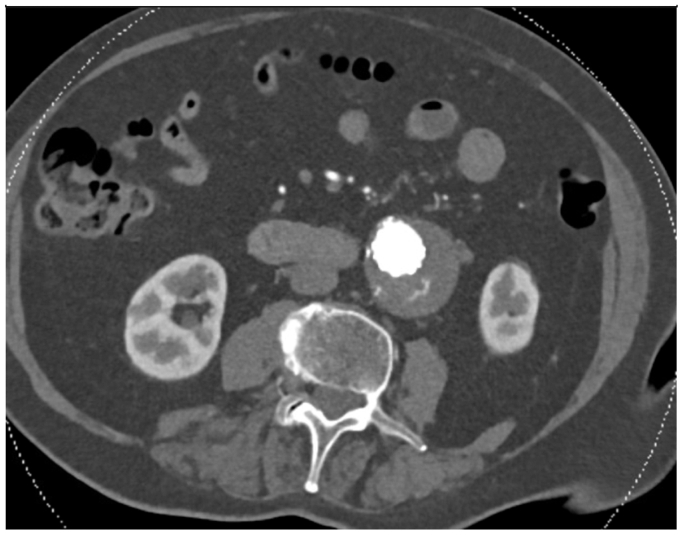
Computed tomography (CT) angiogram showing axial view of type II endoleak after endovascular aneurysm repair (EVAR).
